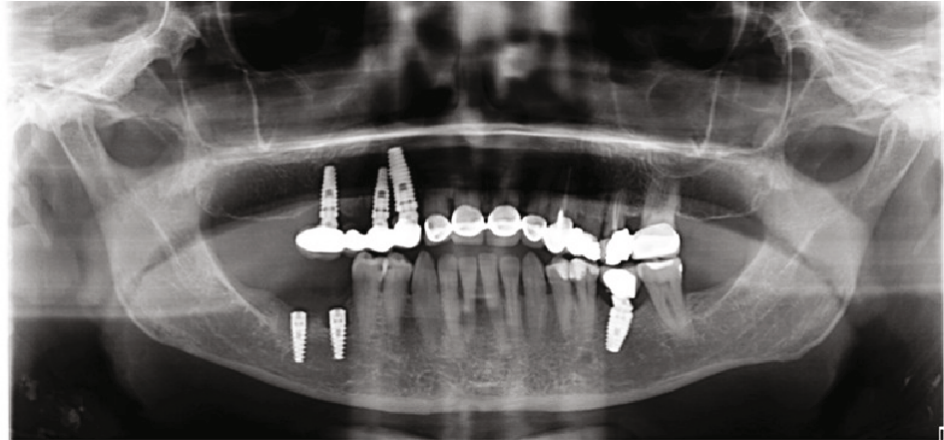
Panoramic radiograph at the 8-year follow-up.
